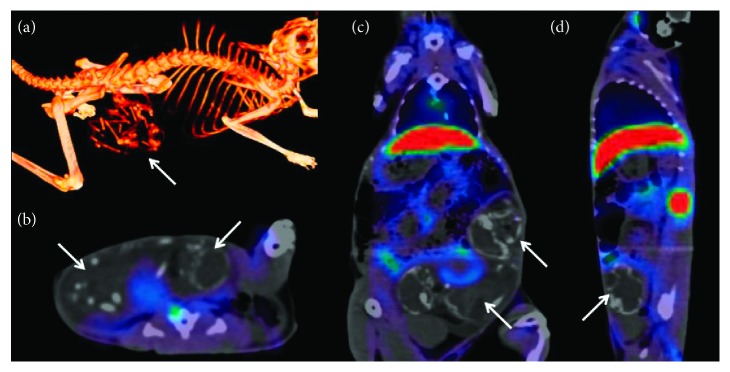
(a) Two fetuses identified in a pregnant chinchilla (the arrow indicates the fetuses) with 3D volume rendering of bone reconstructed CT scan. A summarized [^11^C]-metformin PET/CT scan is visualized in transaxial (b), coronal (c), and sagittal plane (d) (arrows indicate the two fetuses), demonstrating no metformin uptake in the fetuses.
